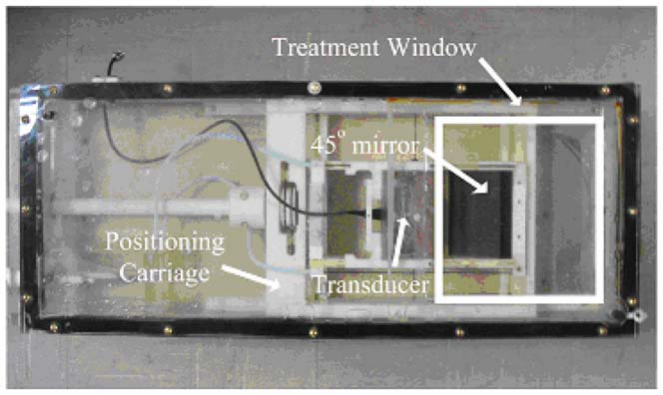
MR-compatible ultrasound transducer and positioning system.
